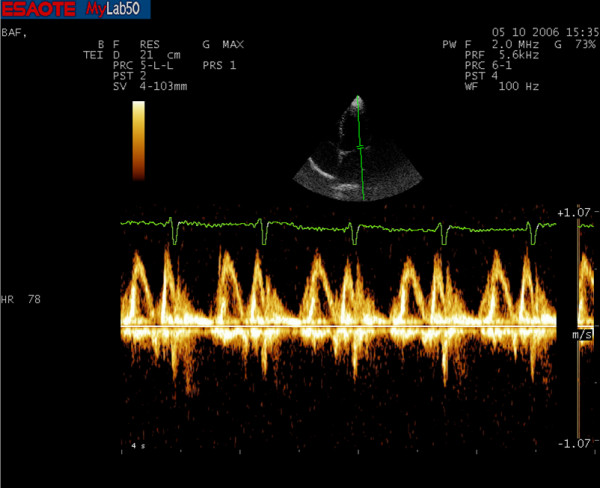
Conventional PW Doppler spectrum of an hypertensive subject.
